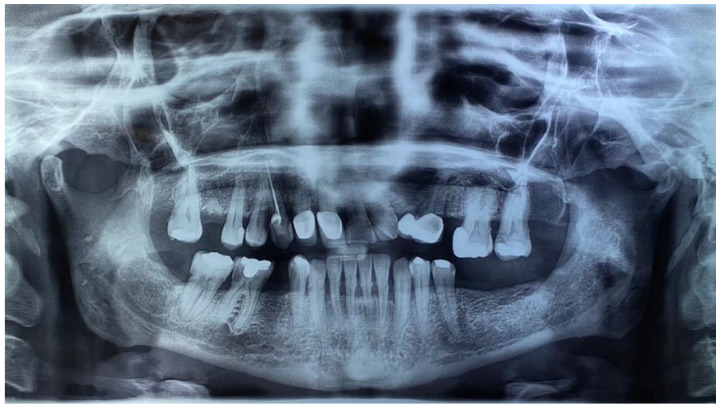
Control orthopantomography at 18 months after surgical intervention.
